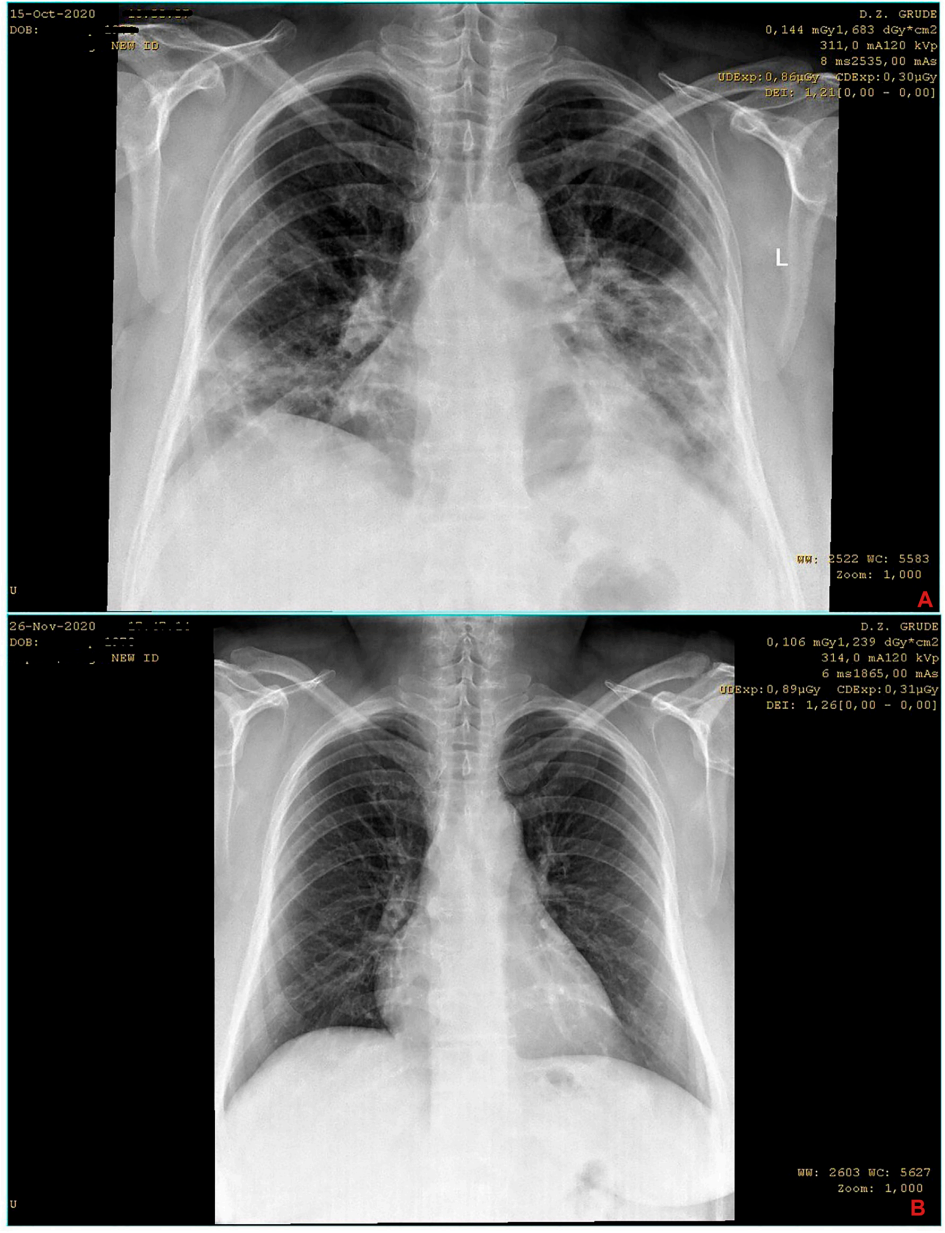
Series of chest X-ray images of COVID-19 patient from case 2. The images are chronologically* arranged in a–z order (the letter mark is indicated in the lower right corner of each image)^§^. *The dates of records are visible in the top right corner of each image. ^§^Identifiable patient’s data are covered.
